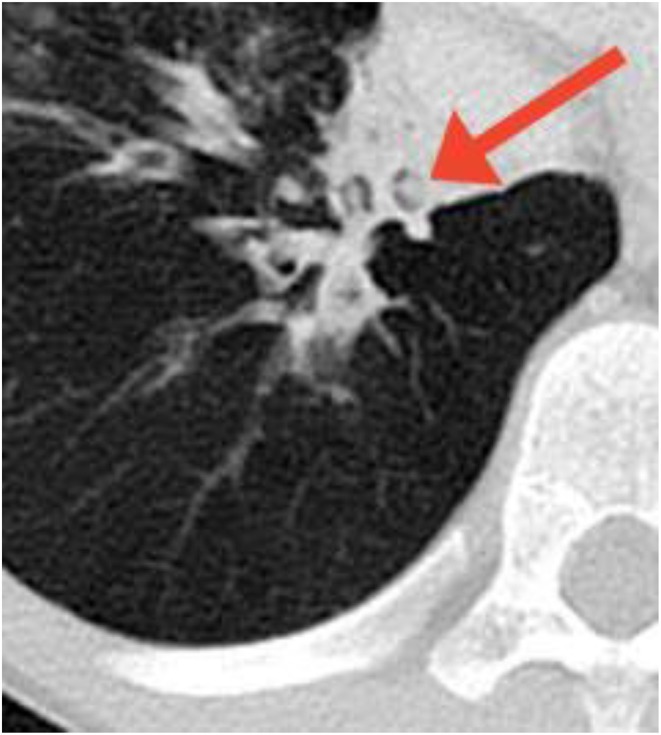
Bronchiectasis in a child with a previously undiagnosed antibody deficiency. Multiple dilated bronchi are evident on CT. Arrow denotes the signet ring appearance of an ectactic bronchus containing a mucous plug and adjacent artery with an abnormal bronchoarterial ratio > 1. Image courtesy David A. Manning, MD, Radiologist, Children's Hospital New Orleans.
